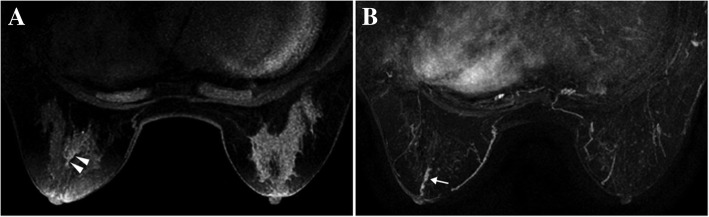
A case of papilloma manifesting as linear enhancement (in 52-year-old woman). a The MIP of the pre-contrast T1-weighted image reveals duct dilation (arrowheads) in the left breast. b The MIP of the subtracted image shows abnormal enhanced lesion with linear distribution (arrow). The pathology is intraductal papilloma with adenosis
